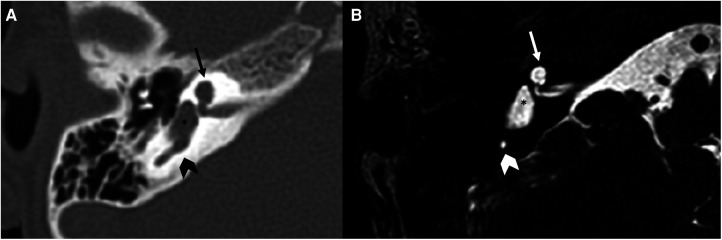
Bone-CT (A) and T2 weighted-MRI (B) imaging of a case of right incomplete partition type I. The anterior and posterior parts of the labyrinth are clearly divided. The anterior part is cystic (arrows), the posterior is dysplastic, although the vestibule (asterisks) is recognizable as well as a part of the posterior semicircular canal (arrowheads).
